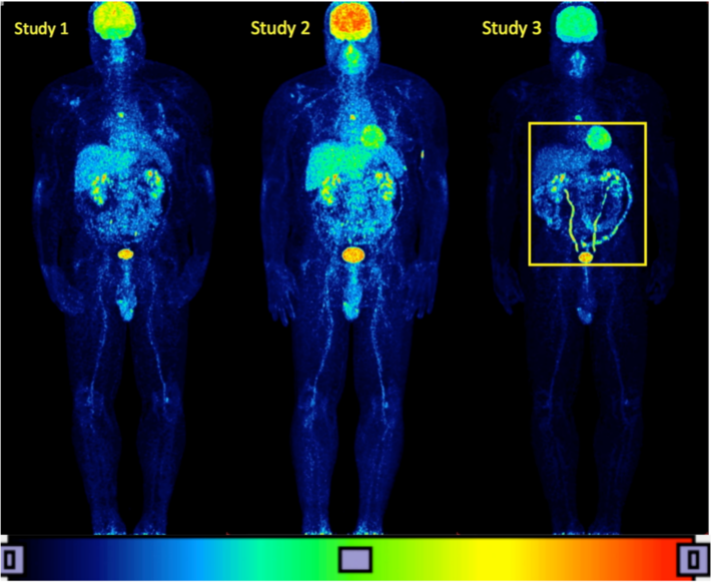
PET/CT images of patient P15 suffering from colitis. The patient presents high uptake of the FDG biomarker not only due to the melanoma metastases but also due to the presence of colitis, not related to the melanoma
